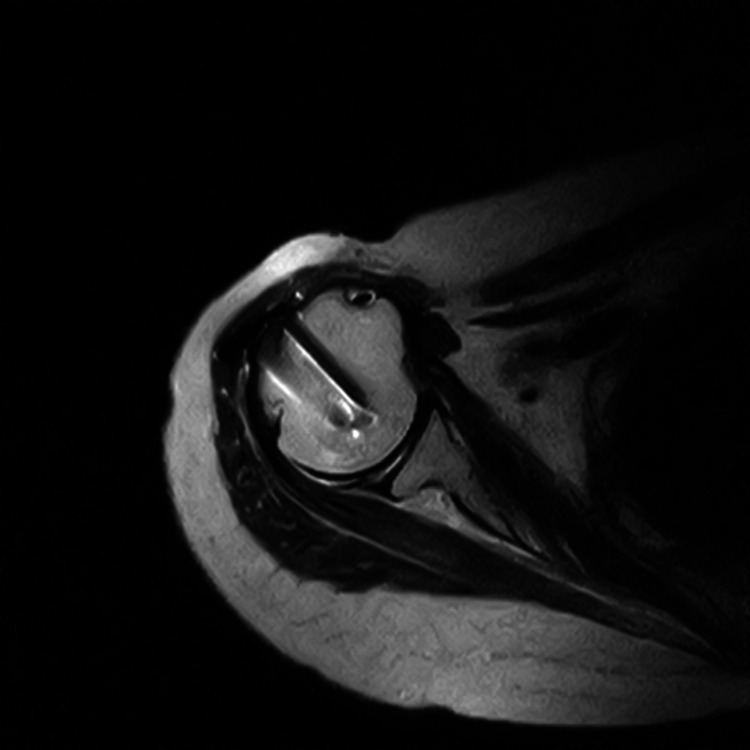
Axial T1-weighted magnetic resonance imaging Magnetic resonance imaging at three months after surgery. The greater tuberosity of the humerus has healed.
